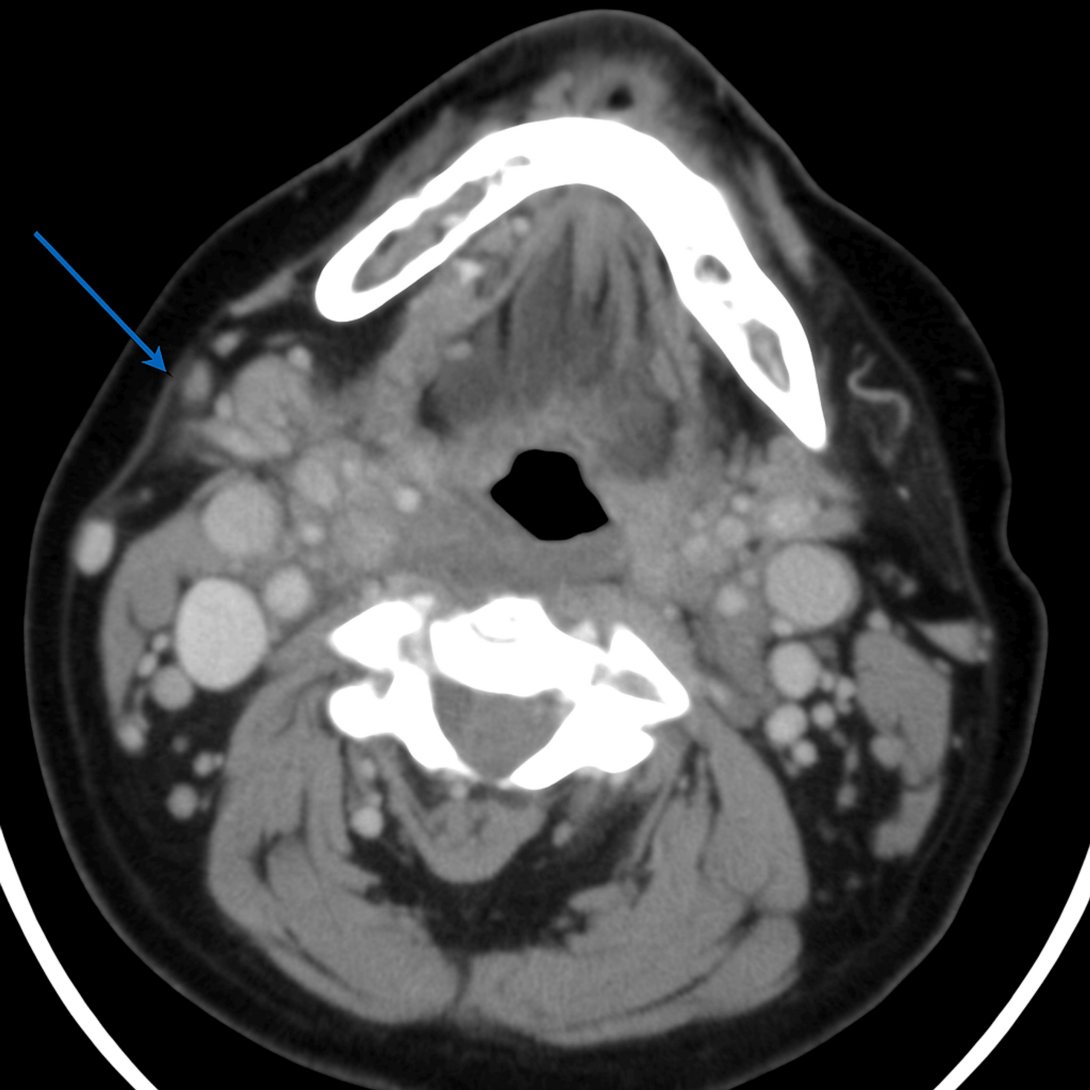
Axial contrast-enhanced CT scan showing minimal subcutaneous stranding at the right submandibular region (blue arrow), indicative of early inflammatory changes consistent with Ludwig's angina in an immunocompromised host.
